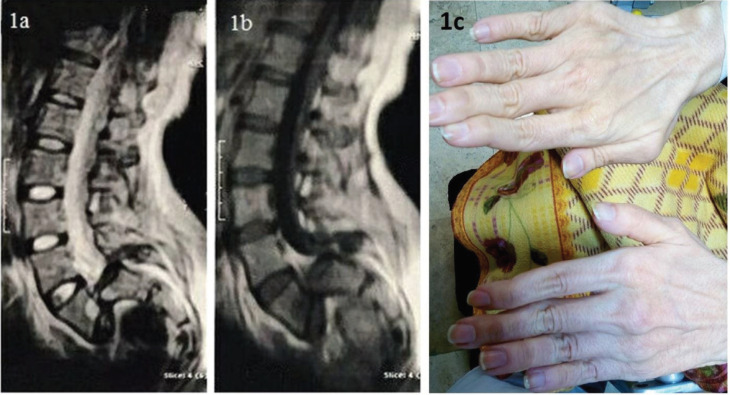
Sagittal T2-weighted (1a) and T1-weighted (1b) MRI of the lumbosacral spine revealed severe spondylolisthesis of L5-S1 vertebrae. The patient had thin and deformed fingers (1c).
